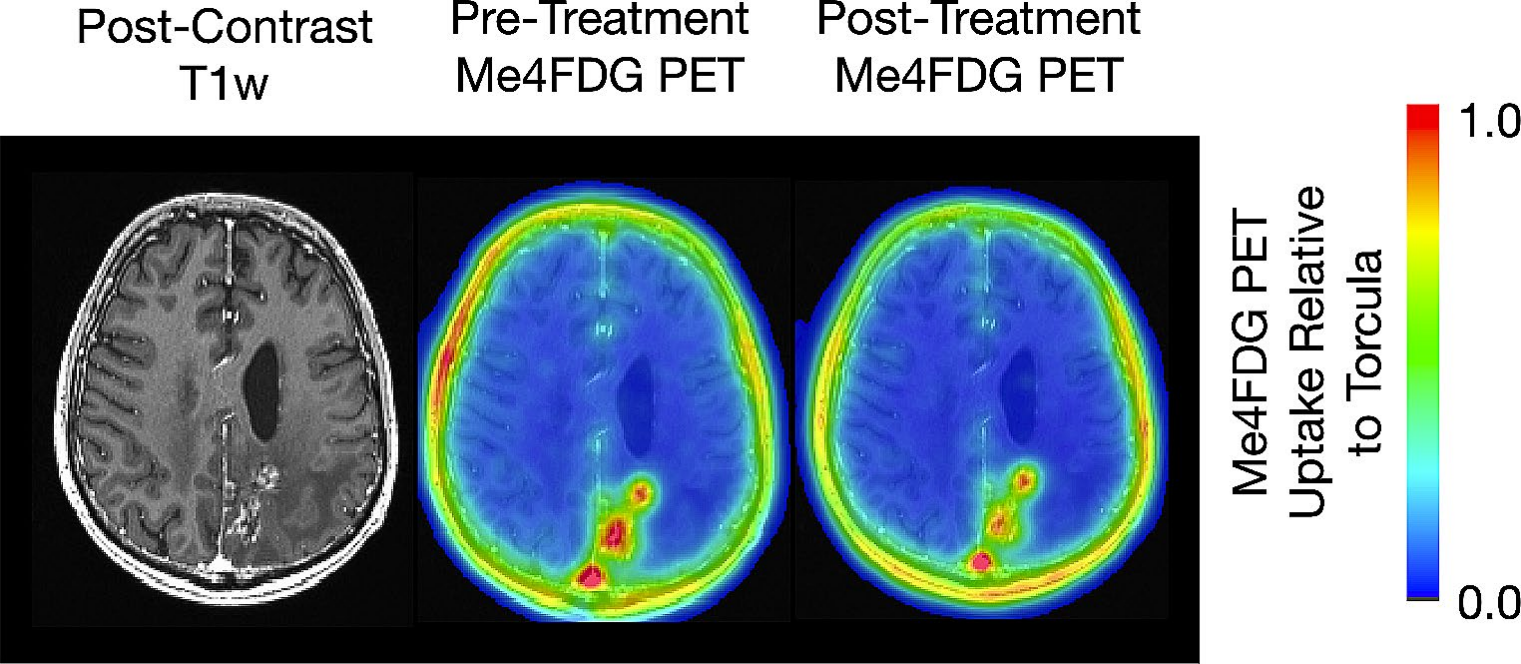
Me4FDG PET scans and a post-contrast T1w MRI of a 52-year-old male patient (SP11) with a recurrent left parietal lobe WHO grade IV glioblastoma. The PET scans were conducted before and after treatment with an oral 100 mg dose of Jardiance. Both the Me4FDG PET scans and the MRI also show the left parietal mass, 4.9 × 3.3 × 4.1 cm, and a second smaller lesion in the left thalamus. The PET images were taken from the sum of the last three frames between 15 and 30 min (see Fig. 2A). No malignancies were detected in whole body CT and Me4FDG PET images
